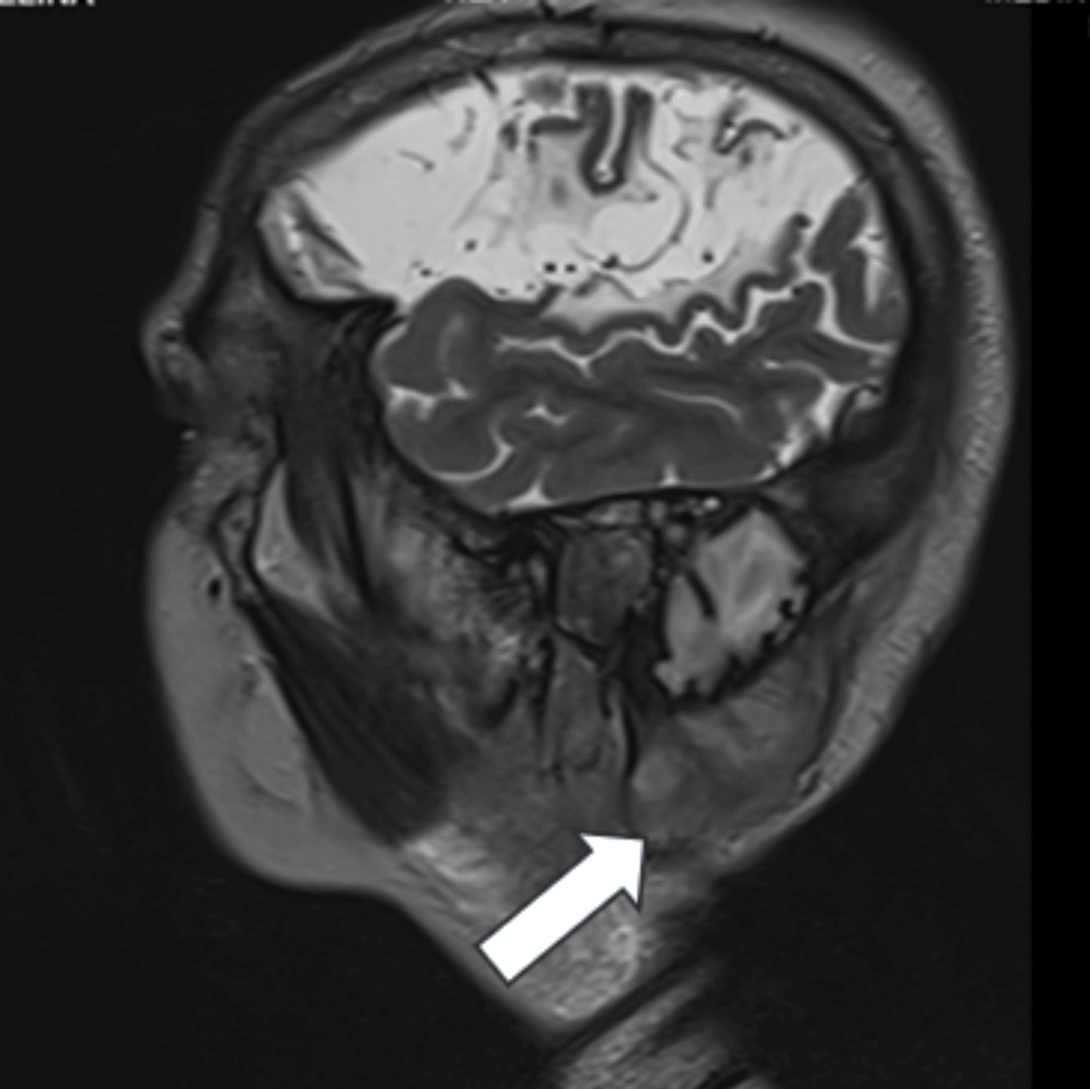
Presence of the Bezold abscess on MRI (white arrow)
